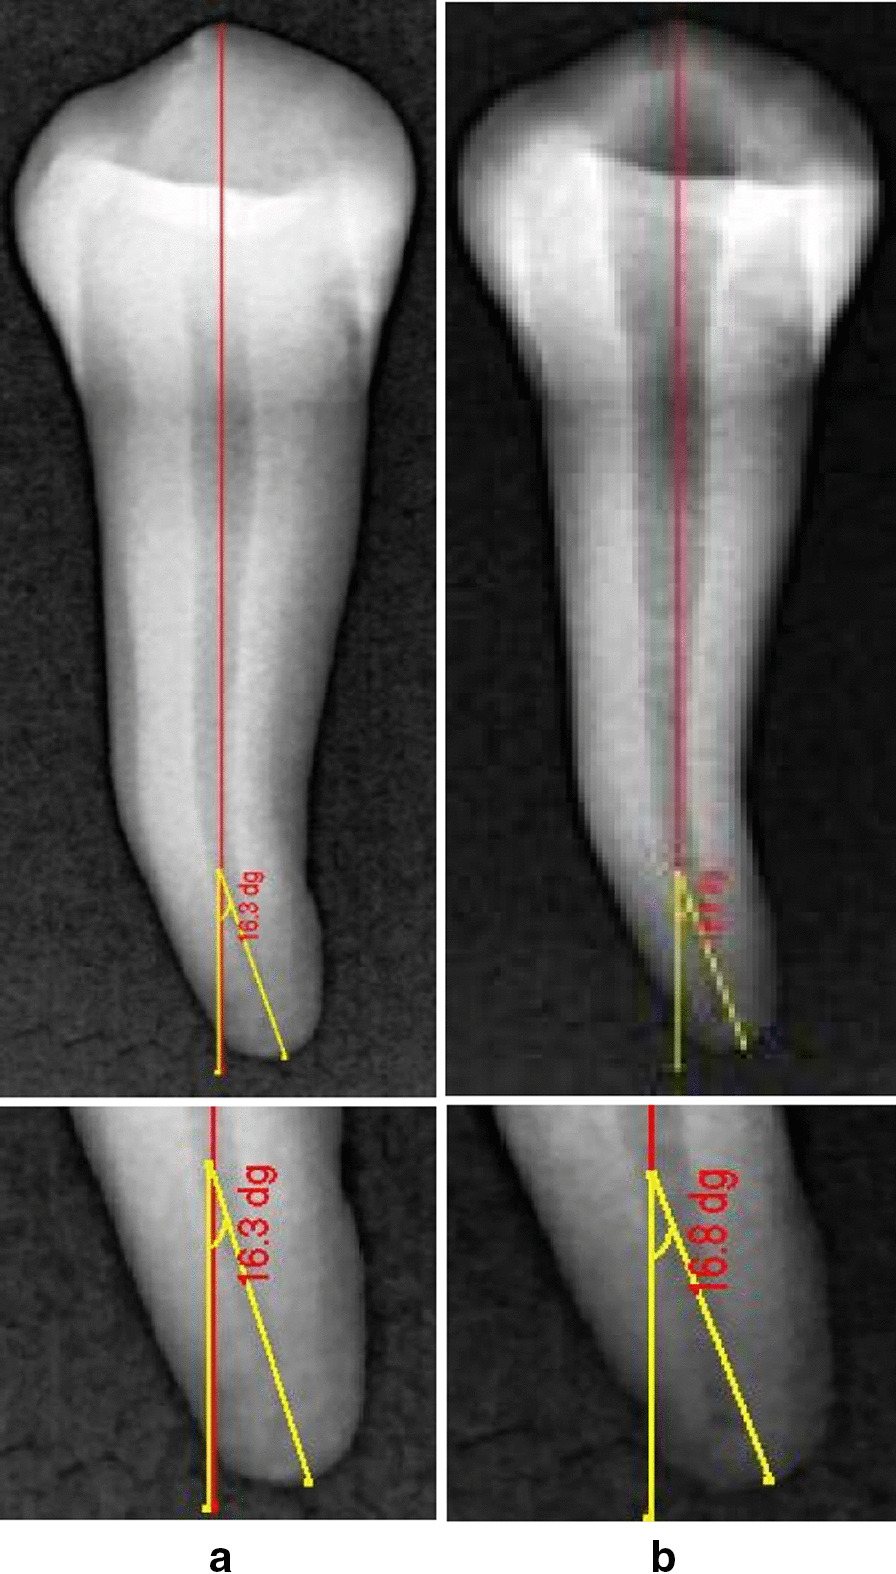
Representative digital periapical images a pre-instrumentation canal curvature angle measurement b post- instrumentation canal axis modification
